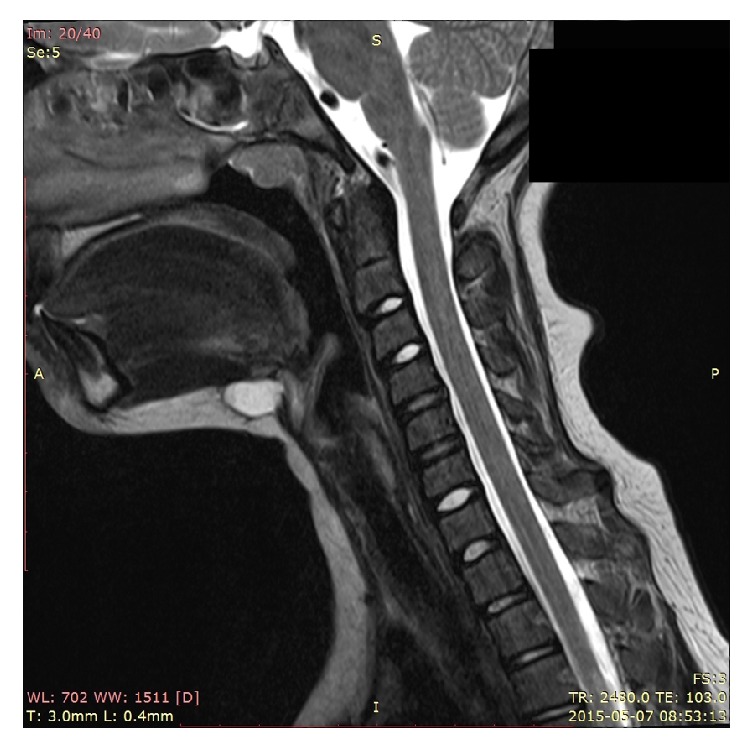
The recurrence of thyrohyoid TGDC in 12-year-old girl in MR imaging.
